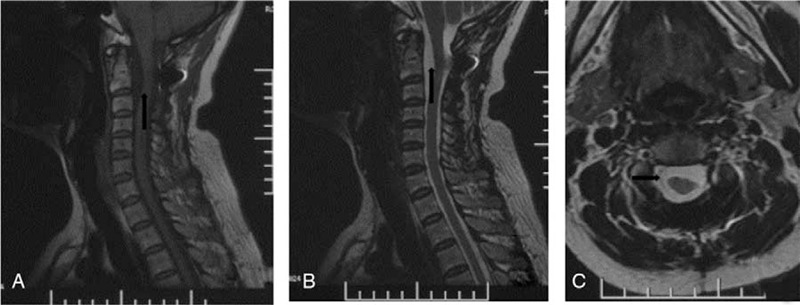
MRI showing that the no recurrence of the tumor 2 years postoperatively. MRI = magnetic resonance imaging.
